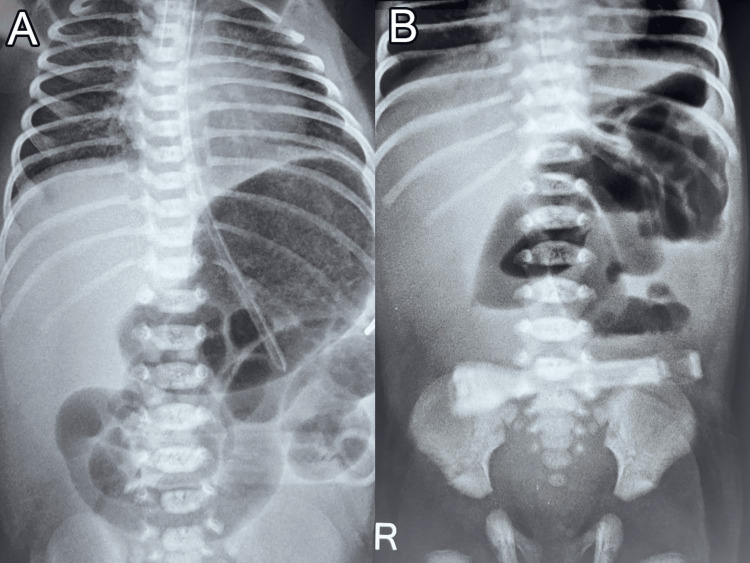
(A) AP view of the abdomen, showing significantly dilated bowel loops. (B) Abdominal X-ray AP view, demonstrating air fluid levels AP: anteroposterior
